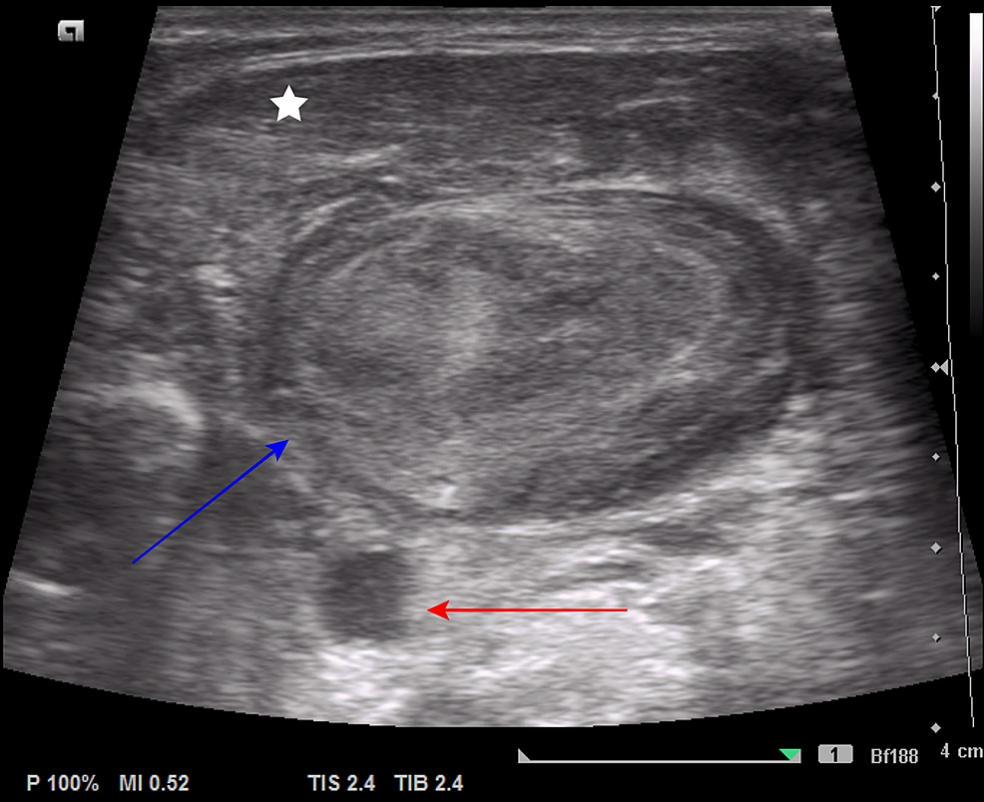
Ultrasound of the left side of the neck It shows the sternocleidomastoid muscle (star), the thrombotic left internal jugular vein (blue arrow), and the left common carotid artery (red arrow).
